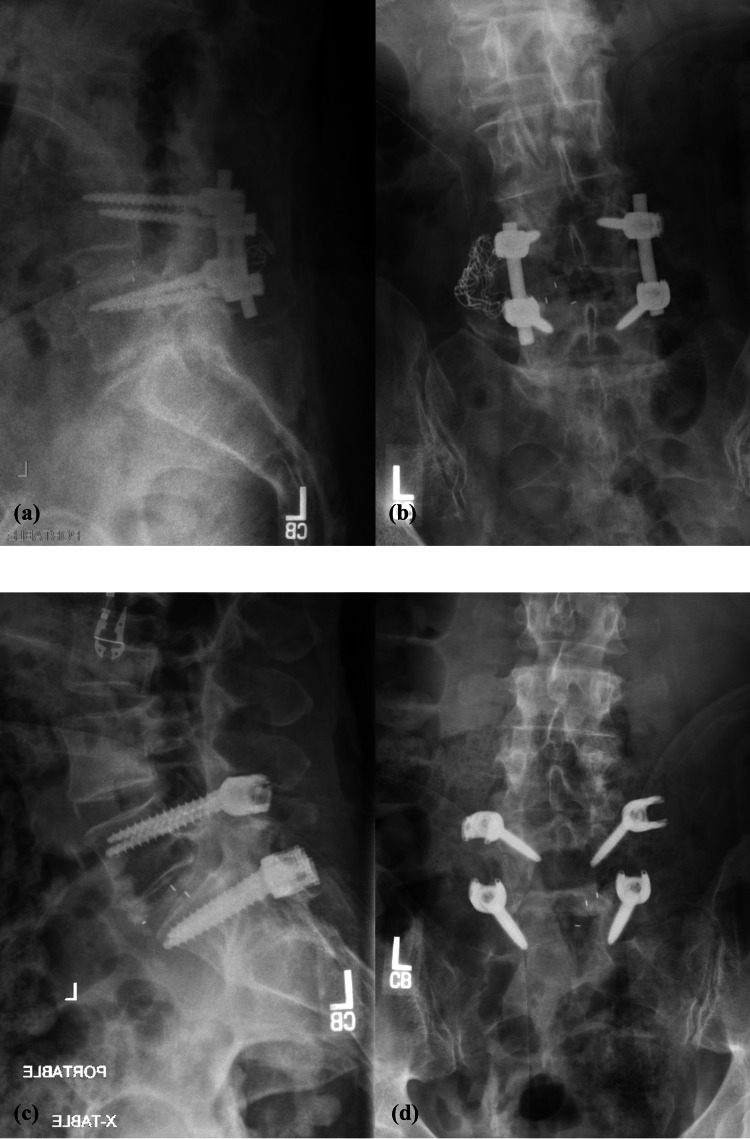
Lateral plus anteroposterior (LAT+AP) radiographs - examples of (a) true positive, LAT (subject 9); (b) true positive, AP (subject 9); (c) false negative, LAT (subject 14); and (d) false negative, AP (subject 14)
